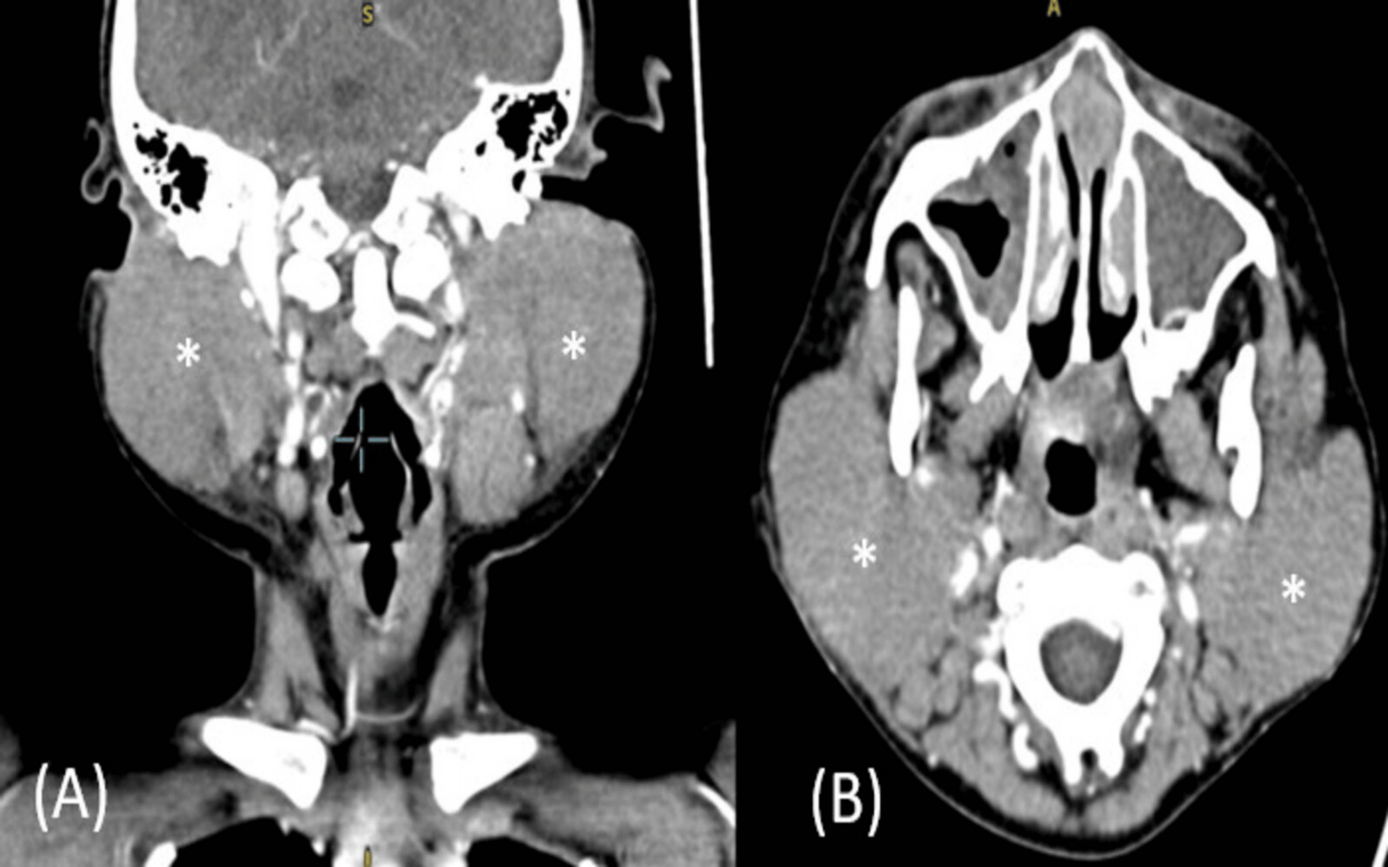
A CECT of the neck showed homogenously enhanced bilateral parotid glands and appeared bulky, but no focal hypodense area with peripheral enhancement was visible to suggest collection. (A) A CECT of the neck sagittal cut with asterisks showed bilateral parotid masses; (B) CECT of the neck axial cut with asterisks showed bilateral parotid masses. CECT: contrast-enhanced computed tomography.
